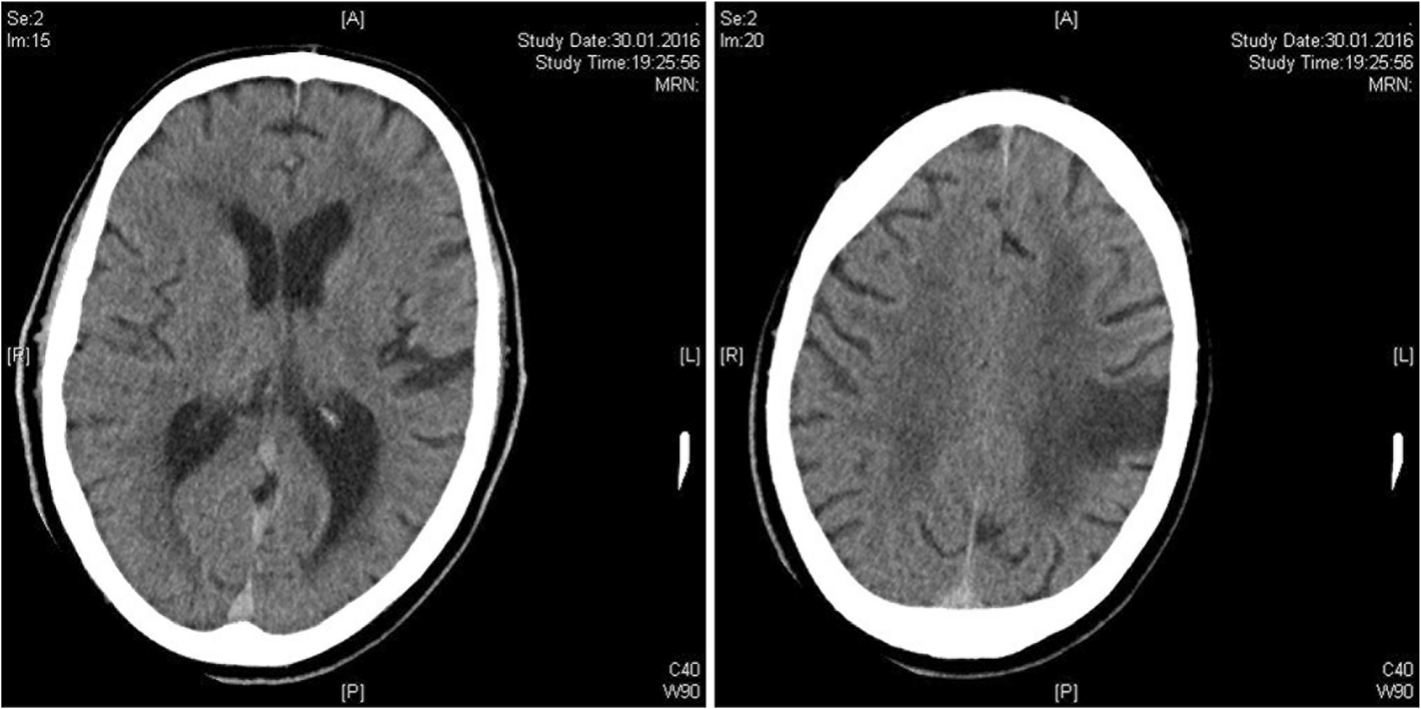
Computed tomographic scan taken upon admission shows no early signs of acute stroke, but displays the residuals of a cardioembolic stroke in the left hemisphere that had occurred in May 2015
